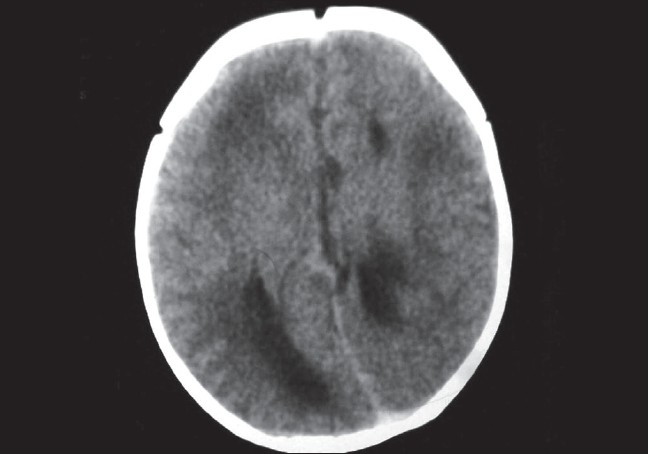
Axial CT scan of ventricles showing dilatation and separation of lateral ventricles with corpus callosum agenesis with colpocephaly
